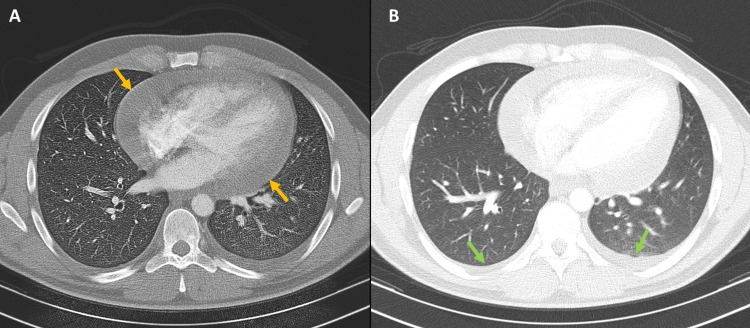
Chest computed tomography (March 9, 2025), axial images. (A) Mediastinal window showing a circumferential pericardial effusion (yellow arrows). (B) Lung window demonstrating bilateral pleural effusions (green arrows).
